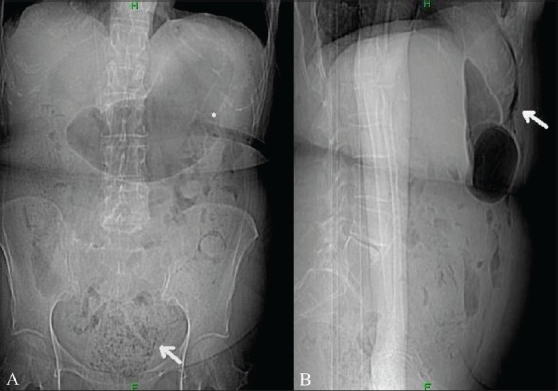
Anteroposterior (A) and lateral (B) scout CT scan images show abundant fecal material and fecalomas in the pelvis (arrow in A) and left colon and pneumoperitoneum (arrow in B)
